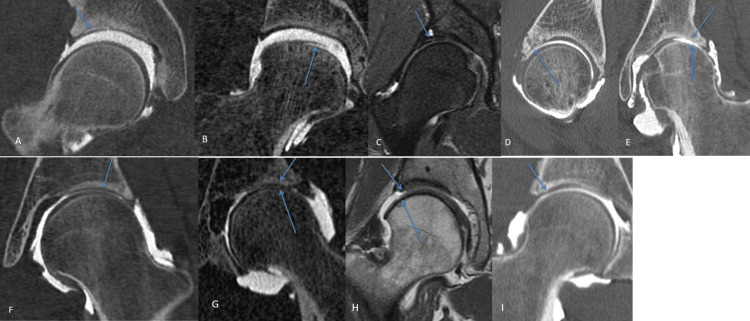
Different kinds of chondral lesions (A) Delamination cartilage lesions in the acetabular or (B) femoral area in computed tomography arthrography (CTA) with traction. (C) Full defect cartilage lesion in magnetic resonance arthrography (MRA) and (D) CTA without traction and (E) with traction. (F) Partial-thickness (<50%) cartilage lesions in CTA without traction and (G) partial-thickness (>50%) cartilage lesions in CTA without traction, (H) MRA, and (I) CTA with traction
